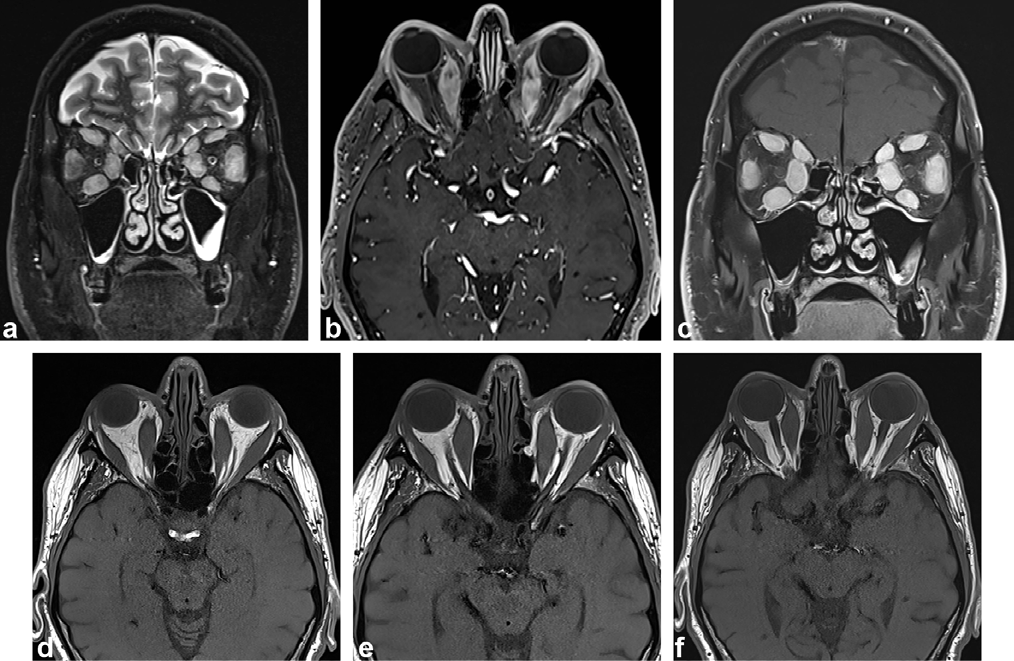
(a–f) Contrast-enhanced MRI of the orbits demonstrates symmetric enlargement of the extraocular muscles. Coronal STIR and axial and coronal contrast-enhanced fat-suppressed *T*
